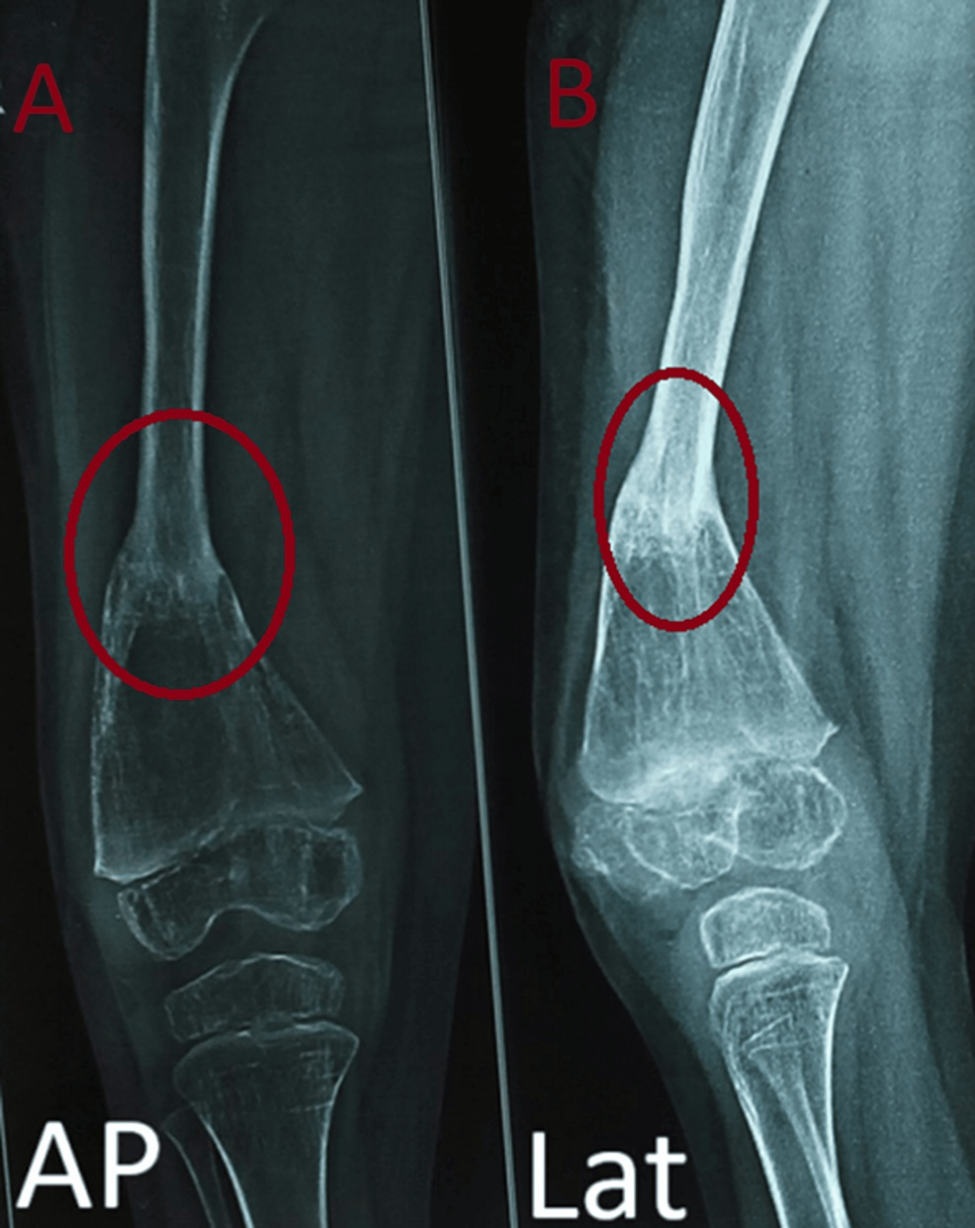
Second-year follow-up radiograph showing satisfactory union at the fracture site A: AP view, B: Lateral view
